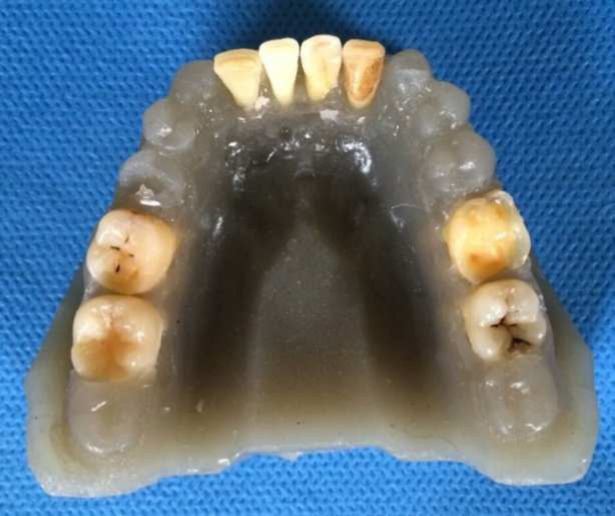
Agar model of mandible for CBCT evaluation
